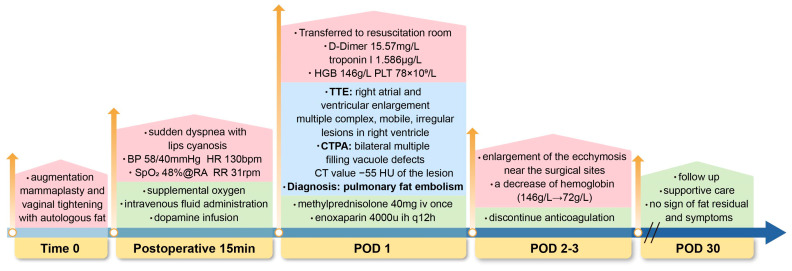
Timeline of diagnosis and management in a case of pulmonary fat embolism after fat grafting. Time 0 (End of surgery); POD, postoperative; BP, blood pressure; HR, heart rate; bpm, beats per minute; SpO_2_, oxygen saturation; RA, room air; RR, respiratory rate; rpm, respirations per minute; HGB, hemoglobin; PLT, platelet; TTE, transthoracic echocardiography; CTPA, computed tomography pulmonary angiography; HU, Hounsfield units; mg, milligram; iv, intravenous; ih, subcutaneous; u, unit; q12h, every 12 h; g/L, gram per liter.
